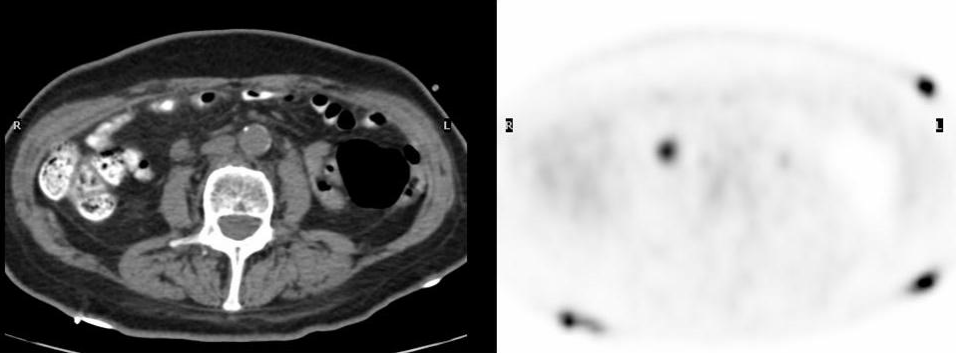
FDG PET-CT images demonstrate a 1.3 cm aortocaval lymph node with intense uptake consistent with metastatic disease.
